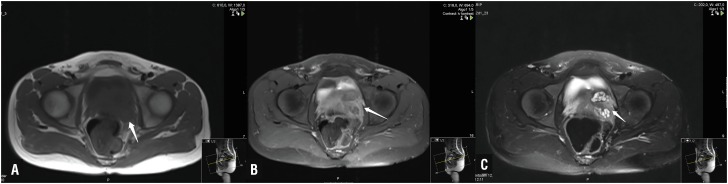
MRI axial images; nodular lesions marked with arrows. A. T1 weighted B. post contrast T1 weighted fat suppressed C. T2 weighted fat suppressed image.
